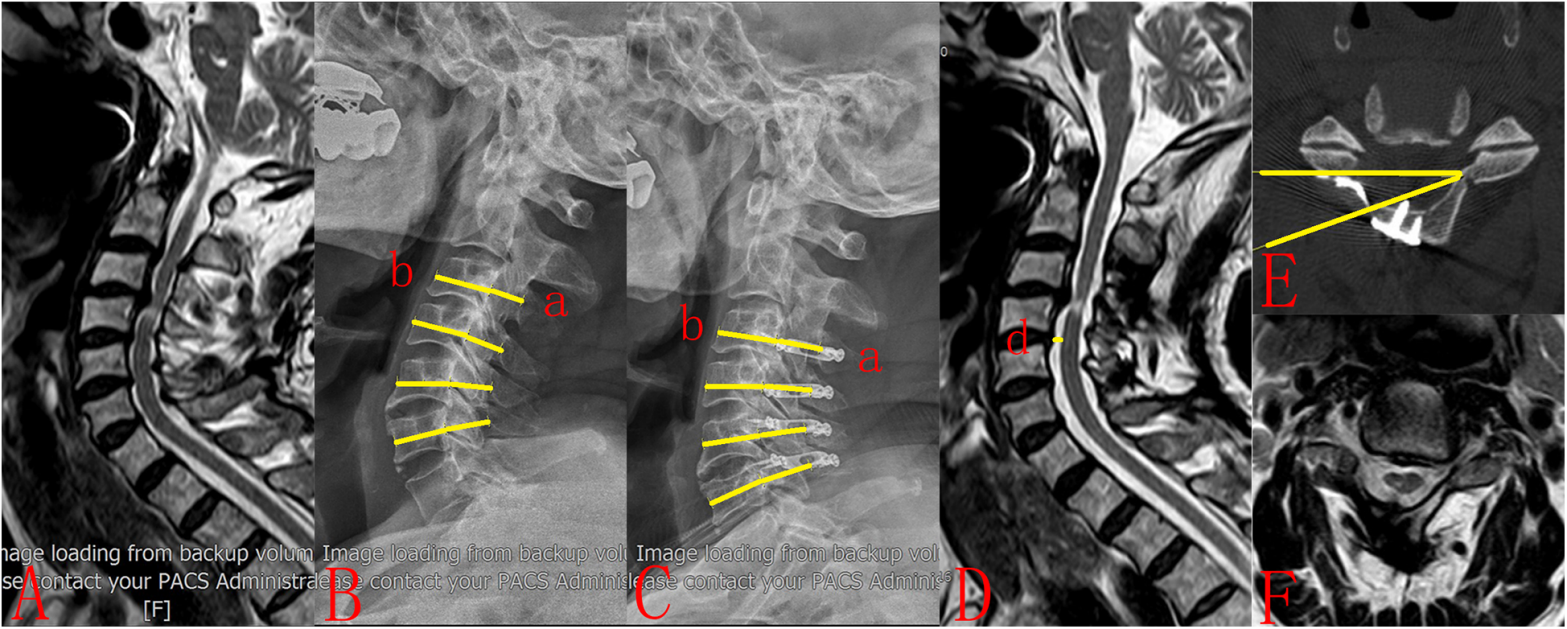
A 62-year-old male patient had been experiencing numbness and asthenia in his limbs for 1 year. (A) Preoperative MRI showed multisegmental spinal cord compression. (B) Preoperative plain radiograph showed cervical canal stenosis (Pavlov ratio = 0.73). (C) The patient underwent cervical unilateral open-door laminoplasty. Centerpiece plates were utilized at the C3-C6 segments (Pavlov ratio = 0.84). (D) Postoperative MRI demonstrated substantial posterior spinal cord drift (*d* = 3.26 mm). (E) Postoperative CT showed that the lamina open angle at C3 was 20.72. (F) Postoperative MRI demonstrated that the spinal cord was completely decompressed.
